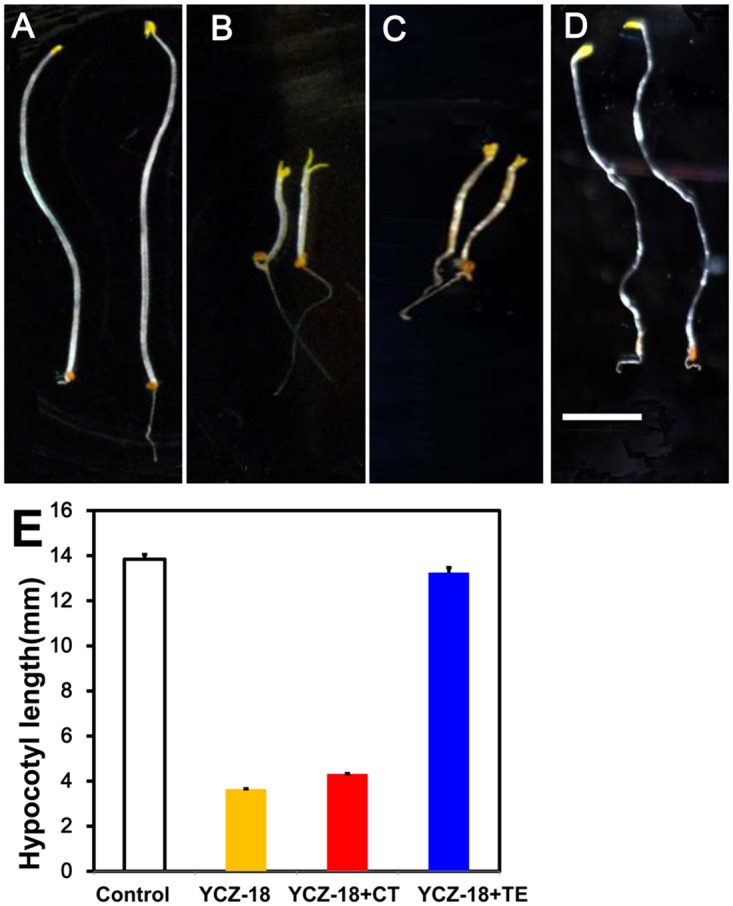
YCZ18-treated Arabidopsis in response to cathasterone (CT) and teasterone (TE). Five-day-old *Arabidopsis* seedlings (8A, 8E, white bar), treated with 0.5 μM YCZ-18 (8B, 8E, yellow bar), treated with 0.5 μM YCZ-18 together with 30 μM cathasterone (CT) (8C, 8E, red bar), or treated with 0.5 μM YCZ-18 together with 10 μM teasterone (TE) (8D, 8E, blue bar). Data are the means ± s.e. obtained from 30 seedlings. Scale bar = 3 mm.
